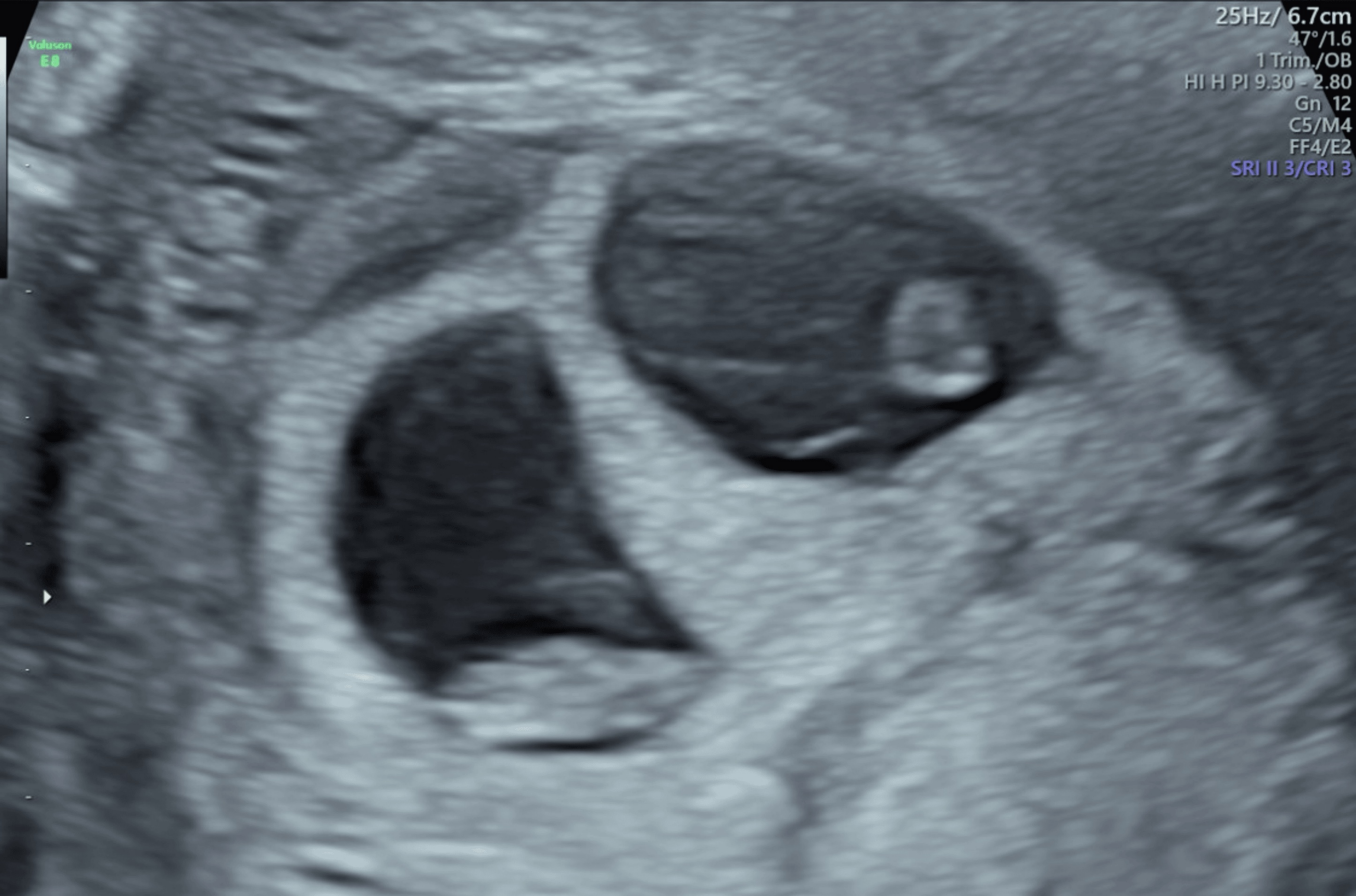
Pelvic ultrasound showing viable twin pregnancy
